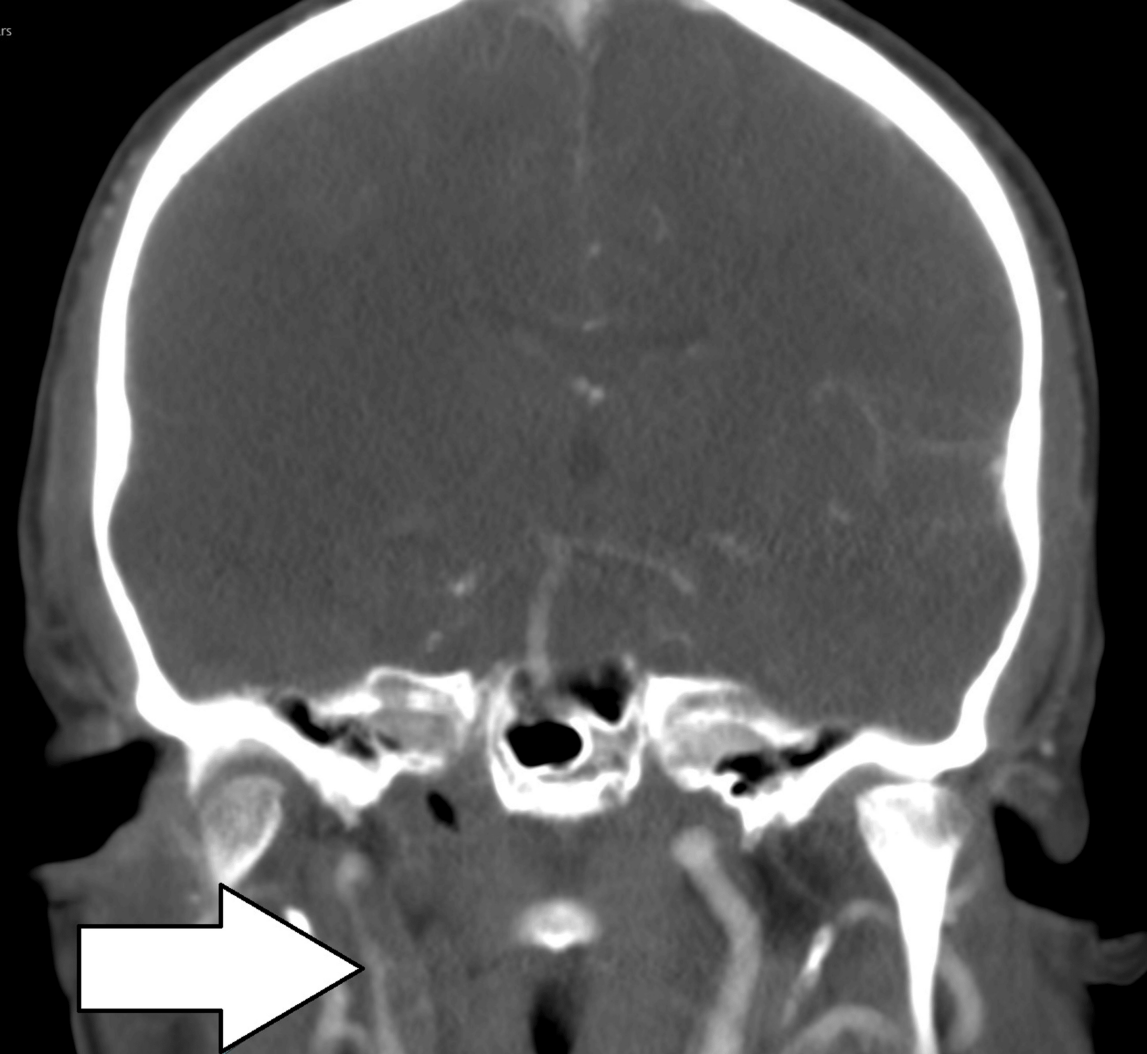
Repeat CT angiogram (coronal view) showing re-canalisation of the proximal right ICA (arrow). CT, Computed Tomography; ICA, Internal Carotid Artery
